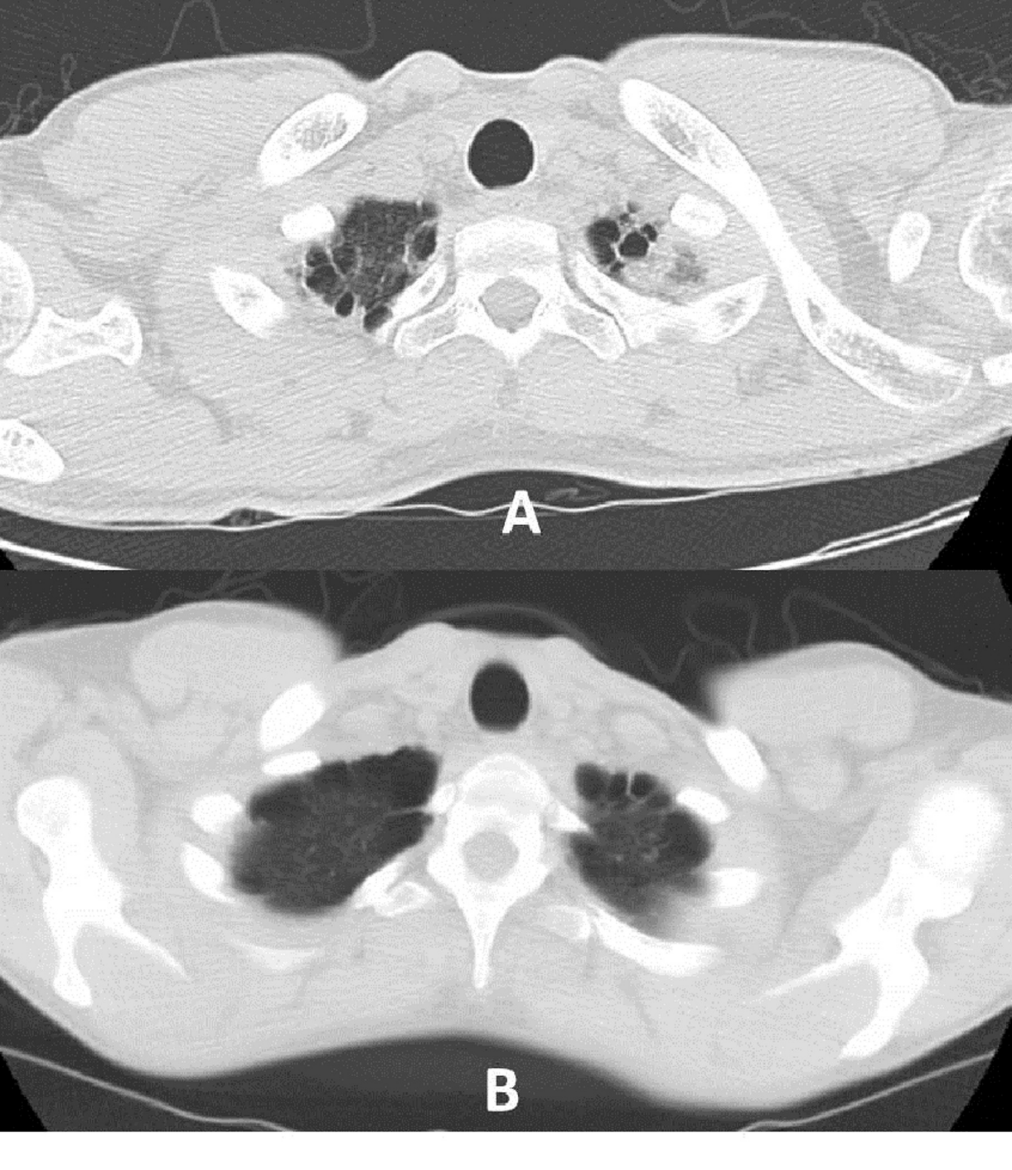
CT scan showing high-grade DSS: A) grade 5 DSS (multiple bilateral blebs), and B) grade 6 DSS (multiple bilateral bullae).
